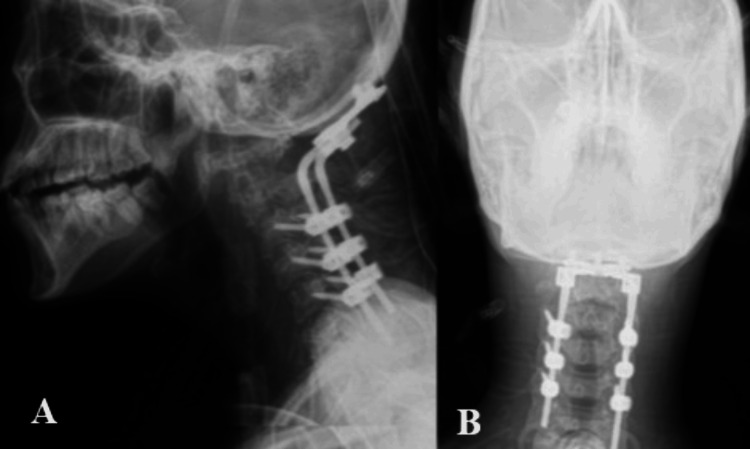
Postoperative X-rays of the cranio-cervical junction A. Lateral view: Postoperative lateral radiograph of the cranio-cervical junction. B. Anteroposterior view: Postoperative anteroposterior radiograph of the cranio-cervical junction.
